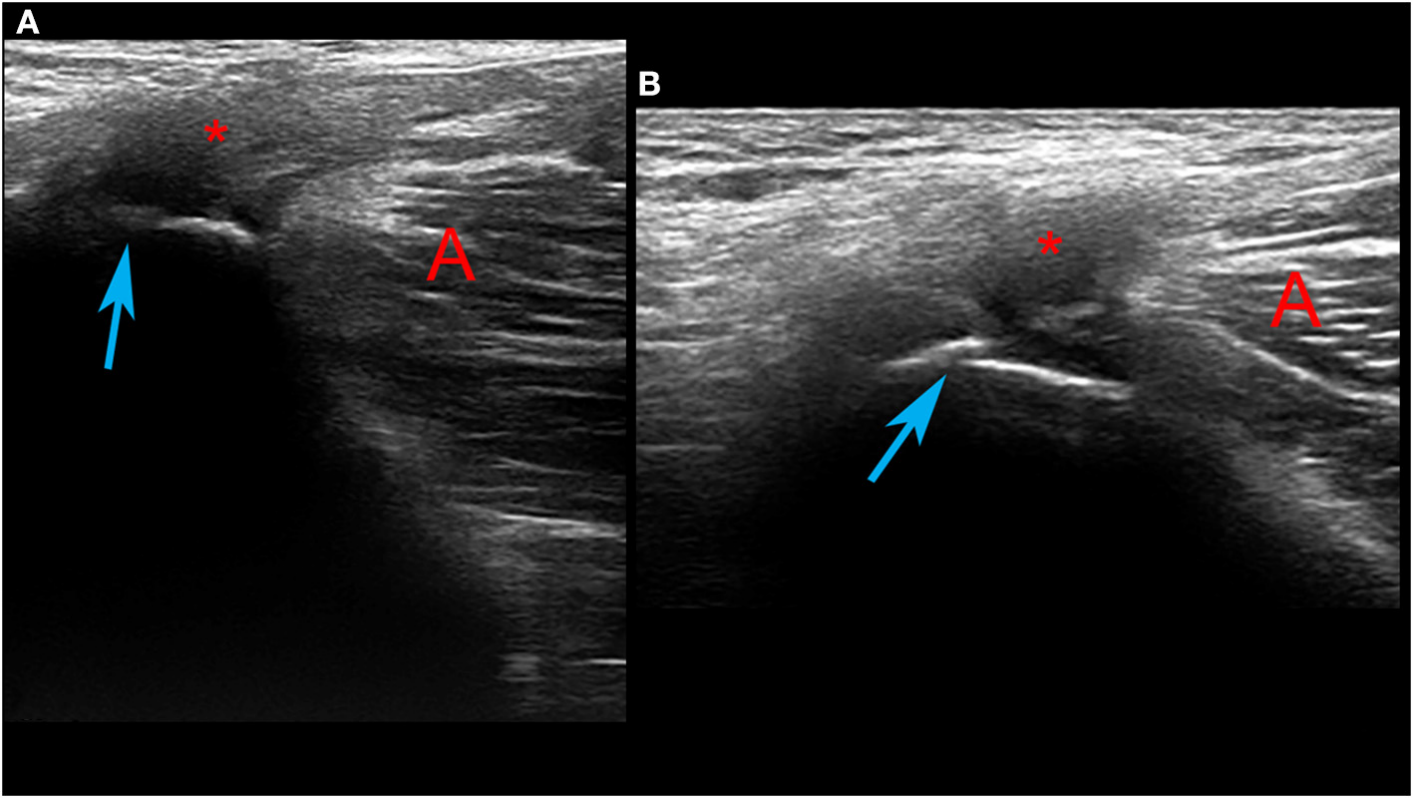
Ultrasound investigation of the pubic area. Functional overload of the adductor insertion (marked with an asterisk) is shown, with an irregularity of the superficial cortical profile (blue arrow) of the pubis. Sections (A,B) provide different planes of view of the pubic functional overload.
